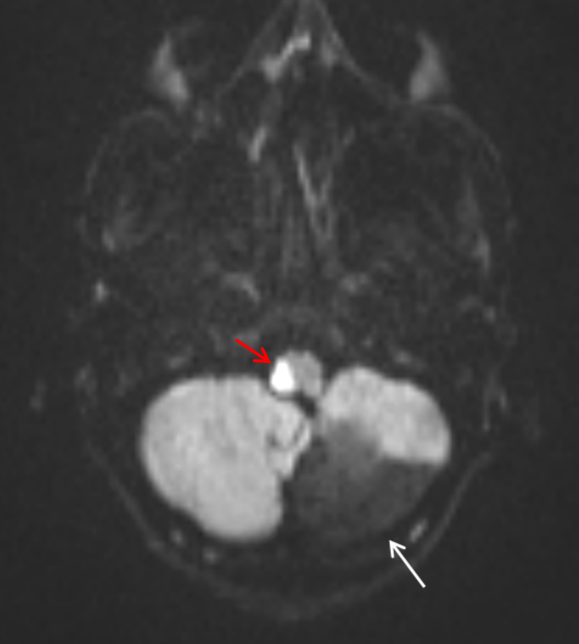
MRI imaging of the brain reveals diffusion restriction in the right medulla oblongata (red arrow) and an old infarction of the left cerebellum (white arrow).
